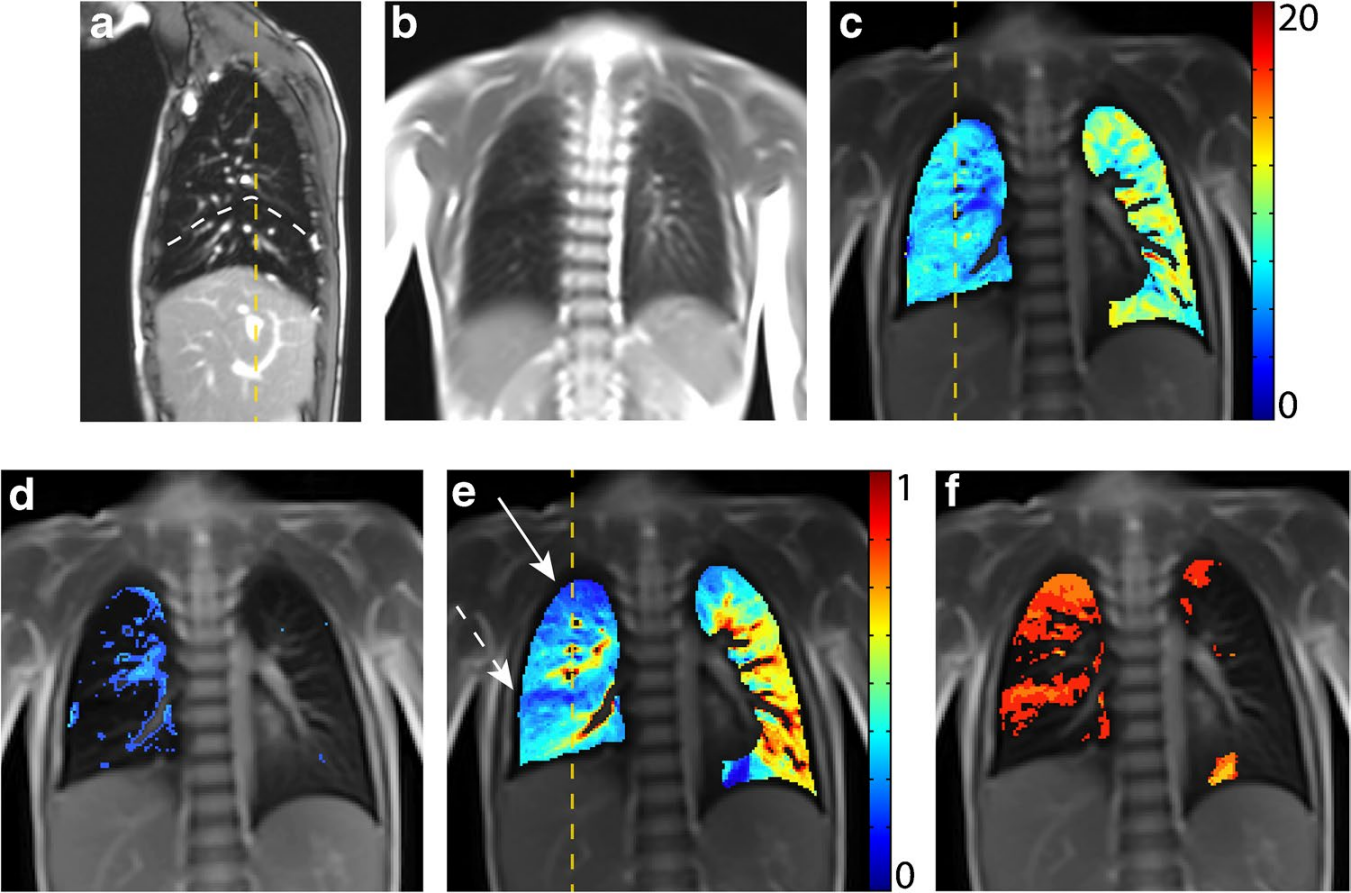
A 5-year-old boy following lobectomy of the right lower lobe for CPAM type 2 and resection of (a) subdiaphragmatic bronchopulmonary sequestration on the right side with an increased lung clearance index of 8.4 turnovers. A sagittal T2-weighted image (a) (repetition time [TR]/echo time [TE] 3.3/1.3 ms) and (b) coronal T1/2-weighted image (TR/TE 1.5/07 ms) demonstrate an expansion of the residual right lung into the lower right thoracic cavity. c-f Coronal functional magnetic resonance imaging maps: (c) coronal fractional ventilation, (d) fractional ventilation defect percentage (brighter blue areas represent reduced function), (e) relative perfusion and (f) relative perfusion defect maps (brighter orange areas represent reduced function) show a fractional ventilation defect percentage (13.6%) within the norm and an increased perfusion defect percentage (24.5%) predominantly in the area of the right middle (*dashed arrow*) and upper lobe (*solid arrow*). *Yellow dashed lines* indicate plane levels. *White dashed* line indicates lung fissure for better visibility. *CPAM* congenital pulmonary airway malformation
